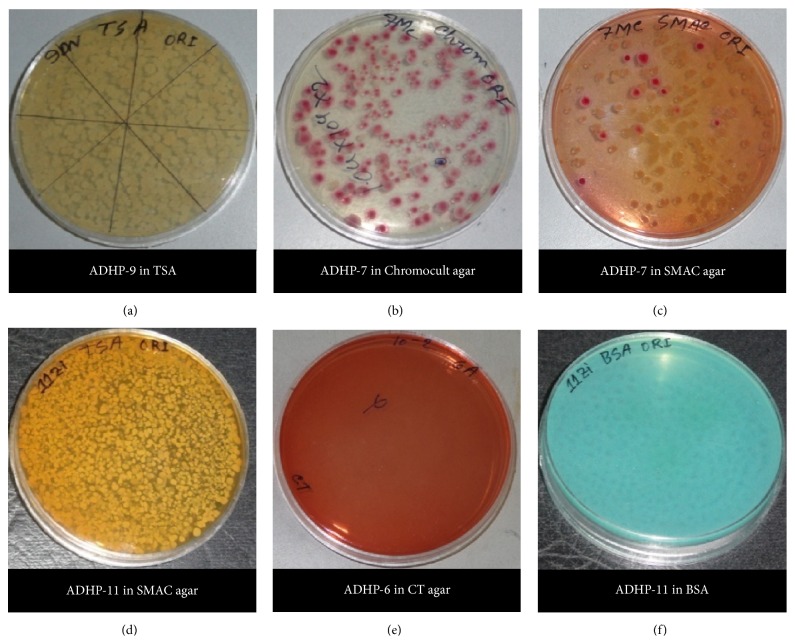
Incubation of antidiabetic herbal preparations (ADHPs) in different agar media for microbial count. (a) ADHP-9 incubated in Tryptic Soya Agar (TSA) plate for total aerobic count, (b) ADHP-7 incubated in Chromocult agar plate for total coliform count, (c) ADHP-7 and ADHP-11 incubated in Sorbitol MacConkey (SMAC) agar plate for total* E. coli* count, (e) ADHP-6 incubated in caprylate-thallous Agar (CTA) plate for* E. coli* 0157 count, and (f) ADHP-11 incubated in Bismuth Sulfite Agar (BSA) plate for* Salmonella* spp. count.
